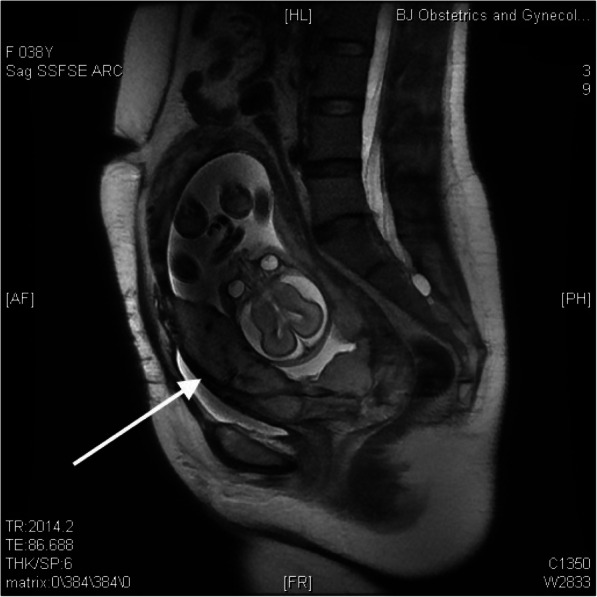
Magnetic resonance imaging at 22 weeks of gestation. Magnetic resonance imaging (MRI) shows the fetus and placenta increta (white arrow)
